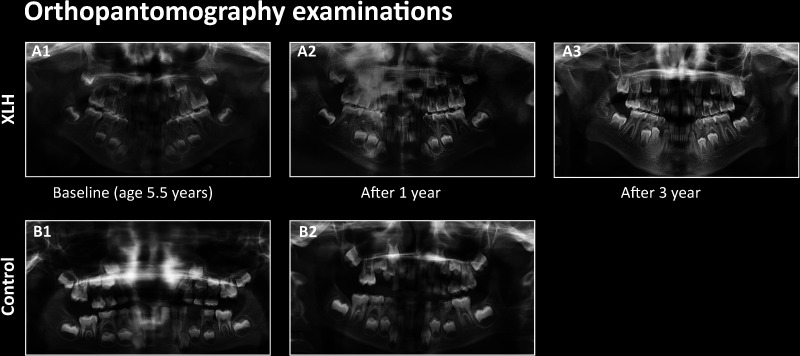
Orthopantomography (OPT) of patient #3 at burosumab initiation (A1), after one year (A2) and after three years of treatment (A3), compared to the OPT of a healthy age-matched boy at baseline (B1) and after one year (B2).
